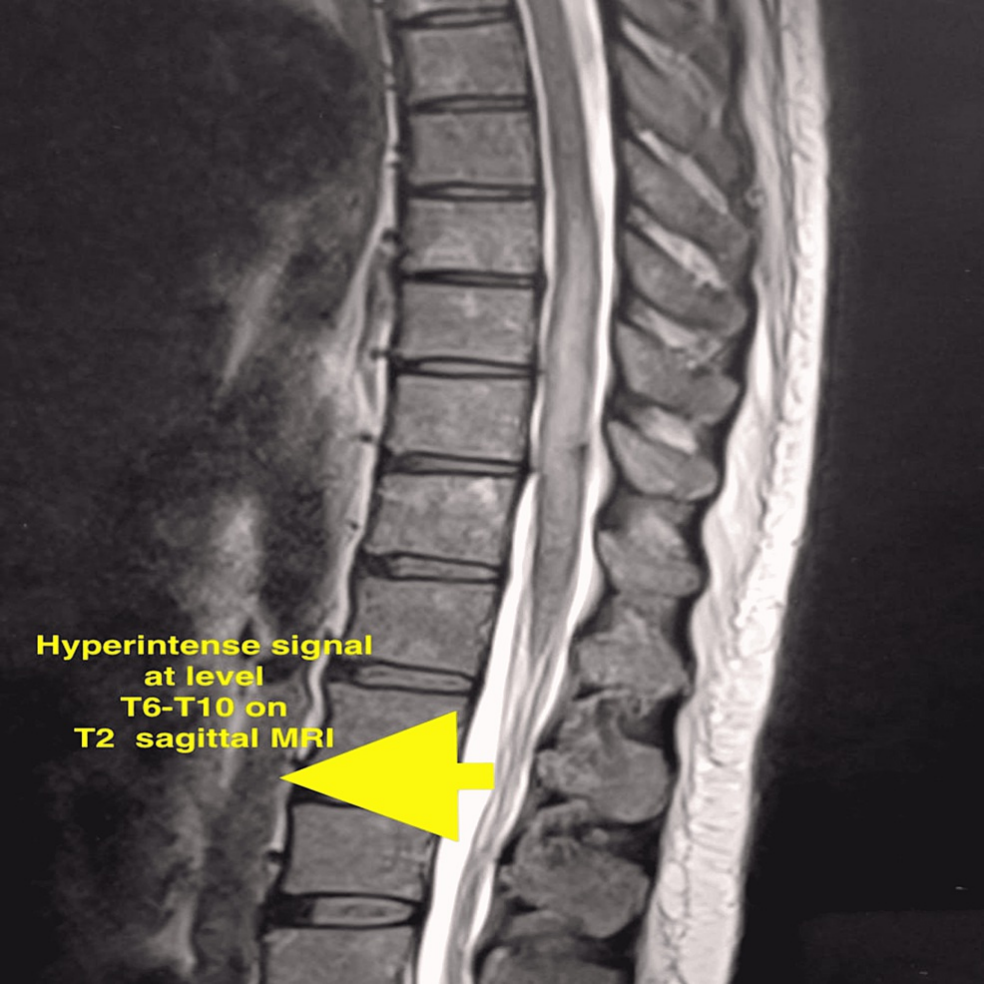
T2 sagittal MRI image of the spine showing hyperintense signal at the T6-T10 levels (arrow)
